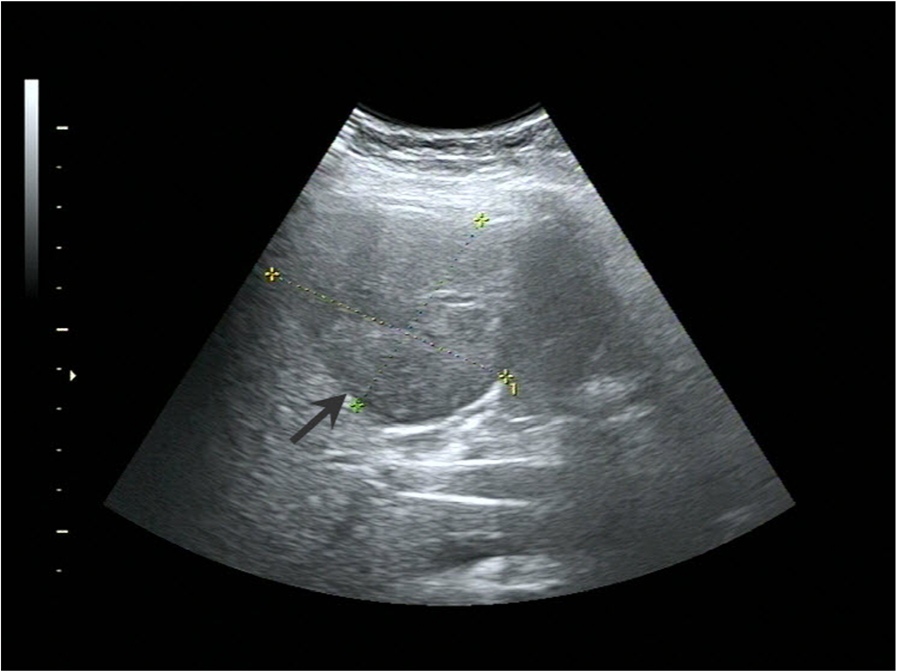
Transverse ultrasound scan of the upper abdomen showing a non-homogeneous, iso-hypoechoic and solid lesion in the right lobe of the liver.
